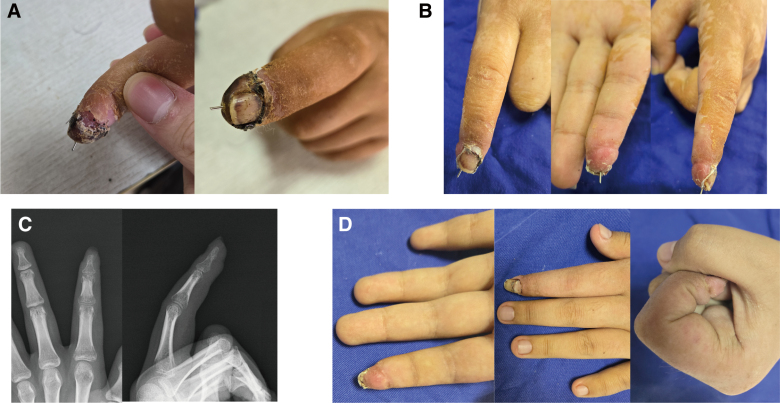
Postoperative photographs after 4 weeks (A) and 8 weeks (B). At 12 weeks postoperatively, radiograph (C) demonstrating complete bony union of the index finger and functional recovery (D) of the replanted fingertip.
